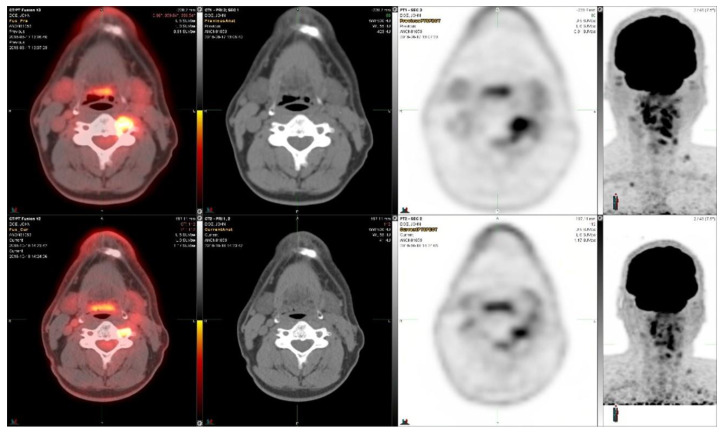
^18^F-FDG-PET/CT images from a medullary thyroid cancer patient who had an RET mutation and was treated with selpercanitib for almost five years. The upper panel demonstrates the situation before selpercanitib treatment; from the left, transaxial PET/CT fusion images, CT images, and PET images at the level of bone metastases (C2–C3), and the furthest right image shows the MIP image from the PET study on the head and neck region. The lower panel shows the situation after two cycles of selpercanitib two months later through similar images at the same level in the transaxial images and in the MIP image shown furthest to the right. In the bone metastasis, the SUVmax decreased from 7.9 to 6.2, corresponding to a 22% decrease.
